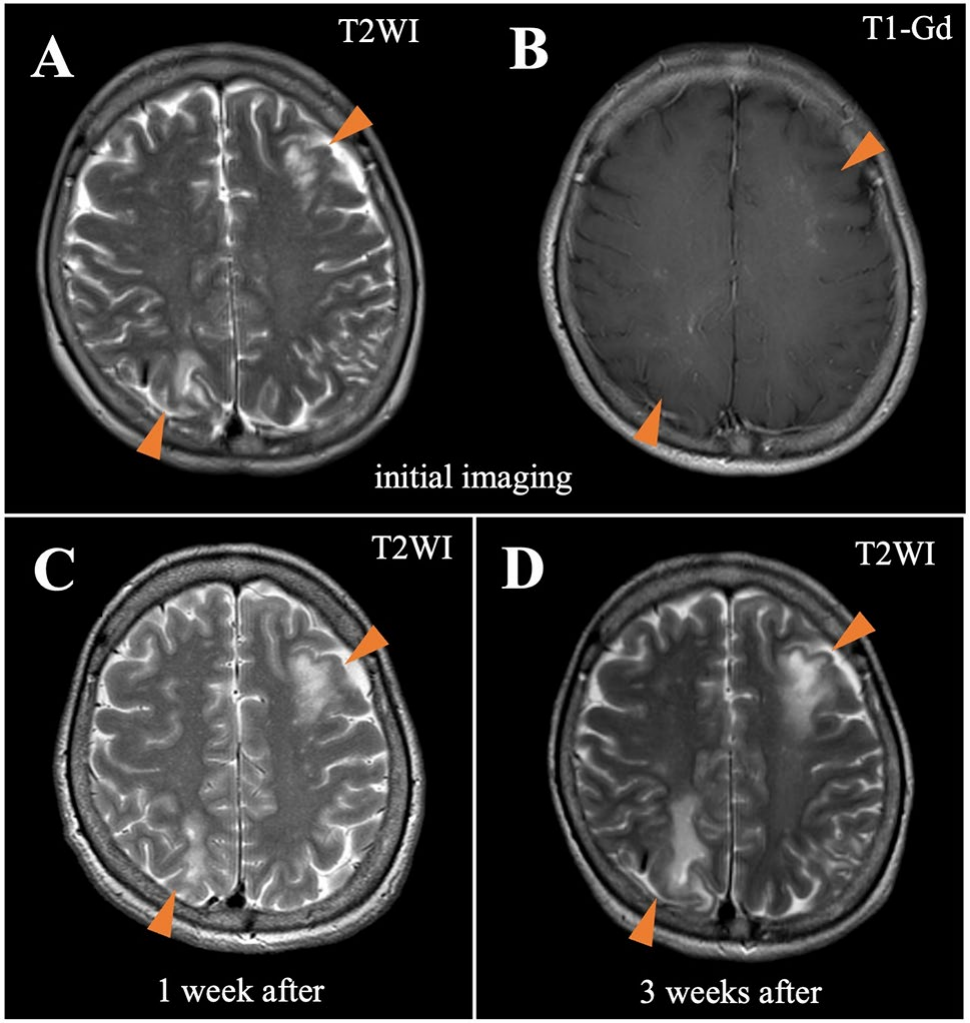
Magnetic resonance imaging of the head. T2WI indicates T2-weigted image; T1-Gd, gadolinium-enhanced T1-weighted image. T2-weighted image at the onset of progressive multifocal encephalopathy (PML) shows asymmetric, cortex-sparing white matter lesions in the left frontal and right parieto-occipital lobes (A). Gadolinium-enhanced T1-weighted image shows partial enhancement in these brain lesions (B). The lesions enlarge weekly, with a confluent pattern (C, 1 week after initial imaging; D, 3 weeks after initial imaging)
